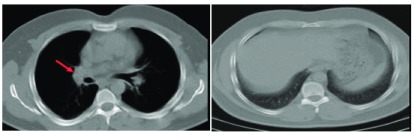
CT scan of patient 6 months after treatment showing decreased size of pulmonary lymphadenopathy and (
